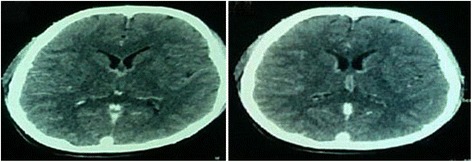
CT scan showed the first result was normal (*left*) but the second showed a smeared bright area in ependymal cells at the lower area of the third ventricle (*right*)
